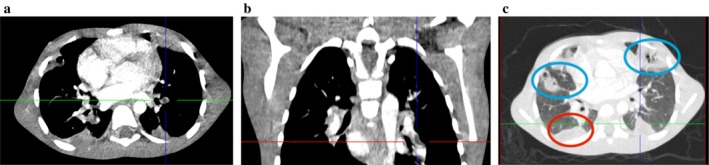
(a) Axial CTPA‐intraluminal filling defect, located centrally within the left lower lobe pulmonary artery (center of crosshair). (b) Coronal reformatted CTPA showing the left lower lobe pulmonary arterial intraluminal filling defect (center of crosshair). (c) Axial CT (lung window) showing wedge‐shaped peripheral consolidation in the left lower lobe (center of crosshair). Similar wedge‐shaped consolidation in the right middle lobe (Red Oval); with cavitating peripheral consolidations in the left upper lobe and right lower lobe (Blue Ovals), consistent with pulmonary infarcts.
